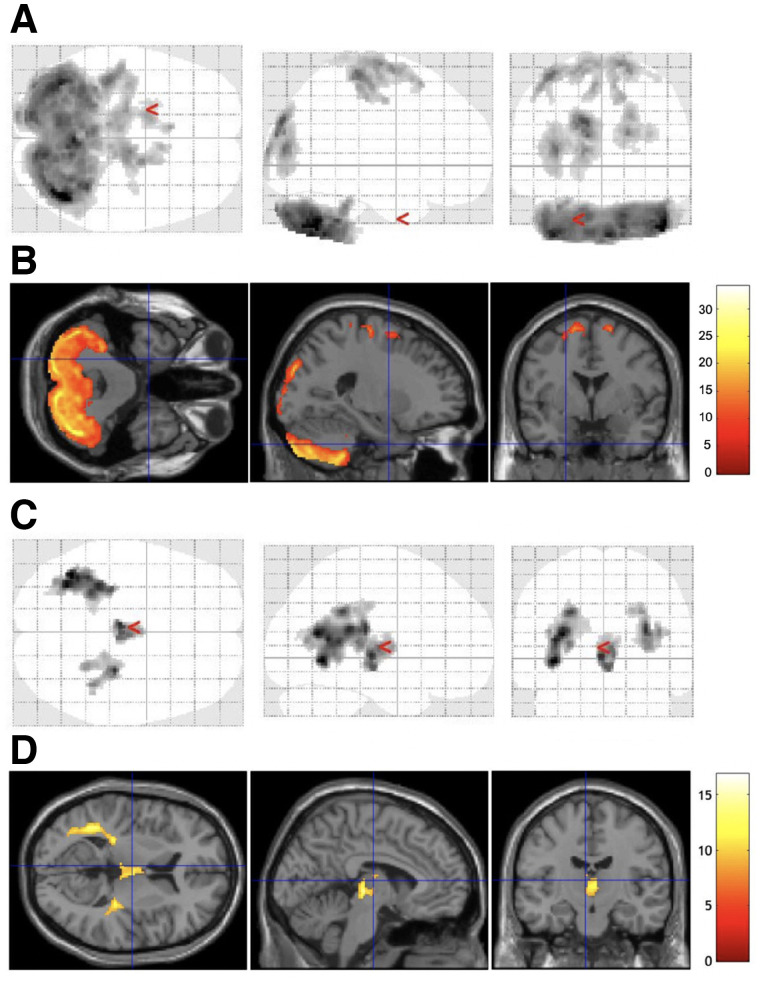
Areas of significant difference in ^18^F-FDG PET images between wbPET scanner and dhPET scanner. Glass brain (a display by SPM, which shows maximum-intensity projection [MIP] of the statistical map in 3 orthogonal planes) (A) and section (B) show higher accumulation in cerebellum, occipital lobe, and around central sulci in images acquired using wbPET scanner than in those acquired using dhPET scanner. Glass brain (C) and section (D) show higher peripheral ventricle accumulations in images acquired using dhPET scanner than in those acquired using wbPET scanner. Scale bar indicates *t* values.
